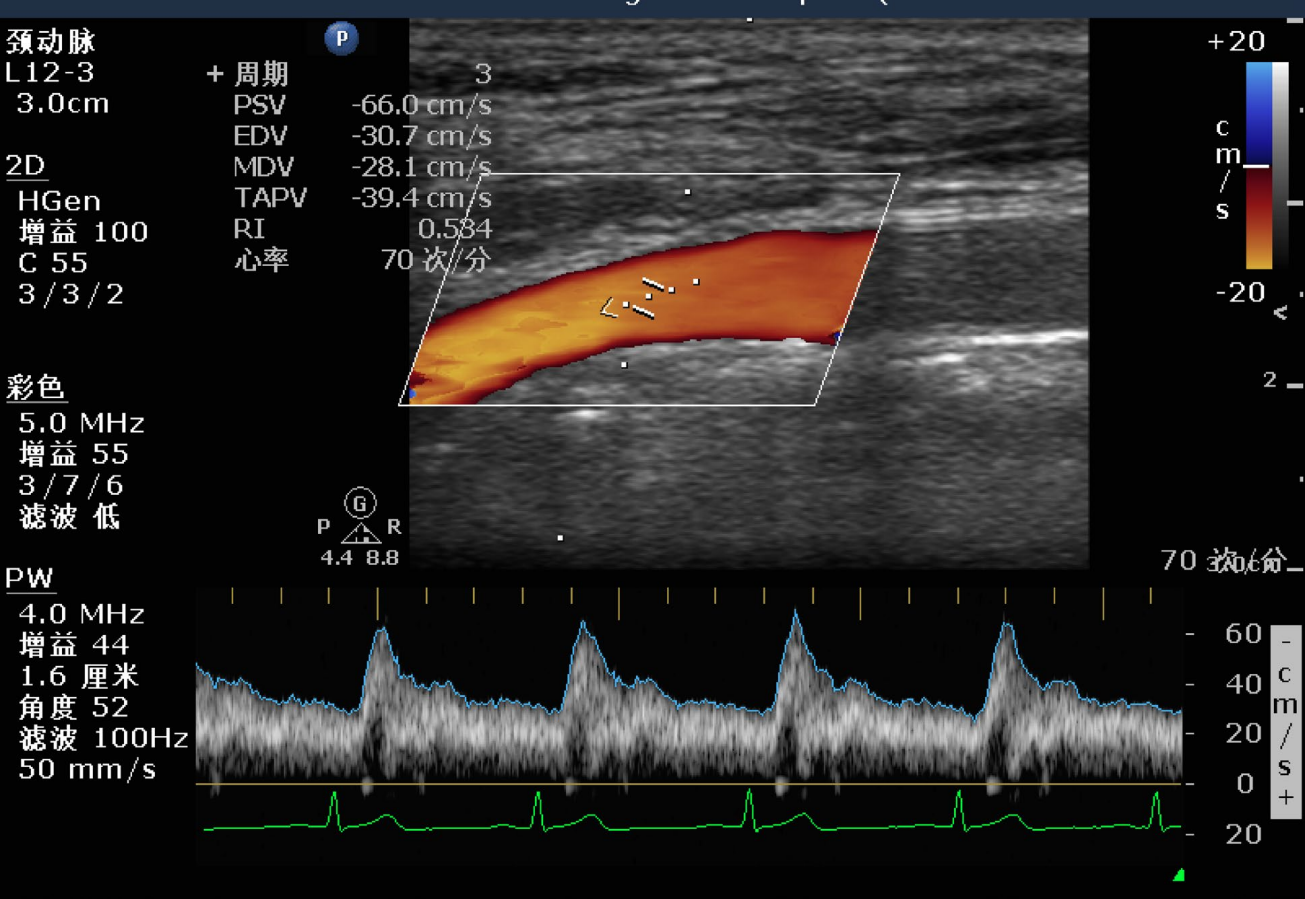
Ultrasound Image of PSV-ICA and EDV-ICA. PSV-ICA=Peak systolic velocity of the internal carotid artery; EDV-ICA= End-diastolic velocity of the internal carotid artery
